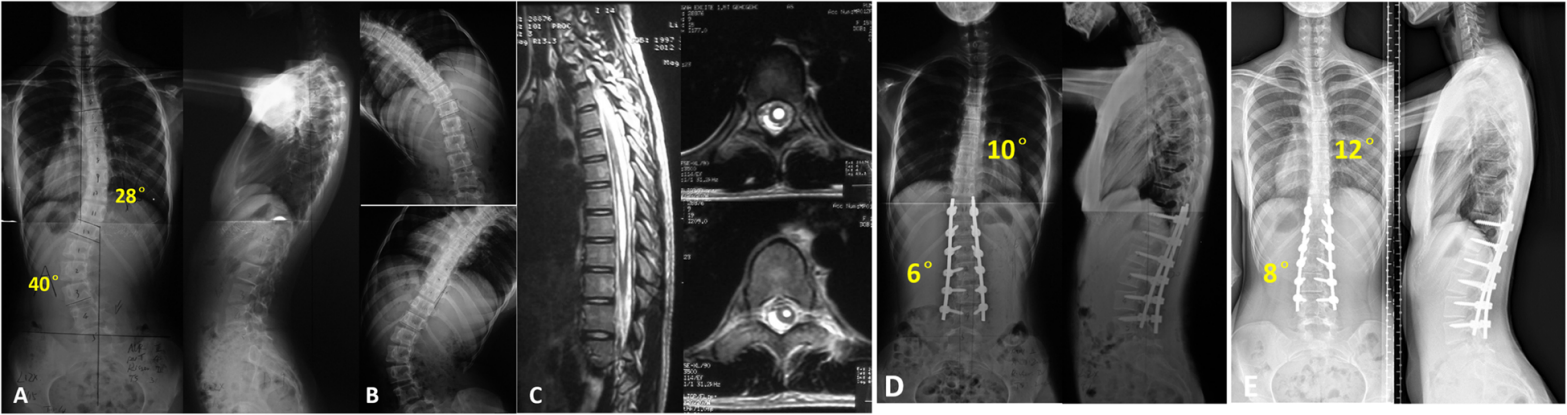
15-year-old girl with a main lumbar curve secondary to syringomyelia. The radiographs demonstrate a lumbar curve of 40° and a thoracic curve of 28° (a). Preoperative bending radiograph shows the high flexibility of major lumbar curve and minor thoracic curve (b). Sagittal and axial views of the syrinx (T5-T8) (c). Postoperative posteroanterior and lateral radiographs following posterior selective lumbar fusion from T11 to L4 demonstrate satisfying correction of syringomyelia-associated scoliosis (d). Radiographs made at three years follow-up (e) show that correction outcome had been well maintained
